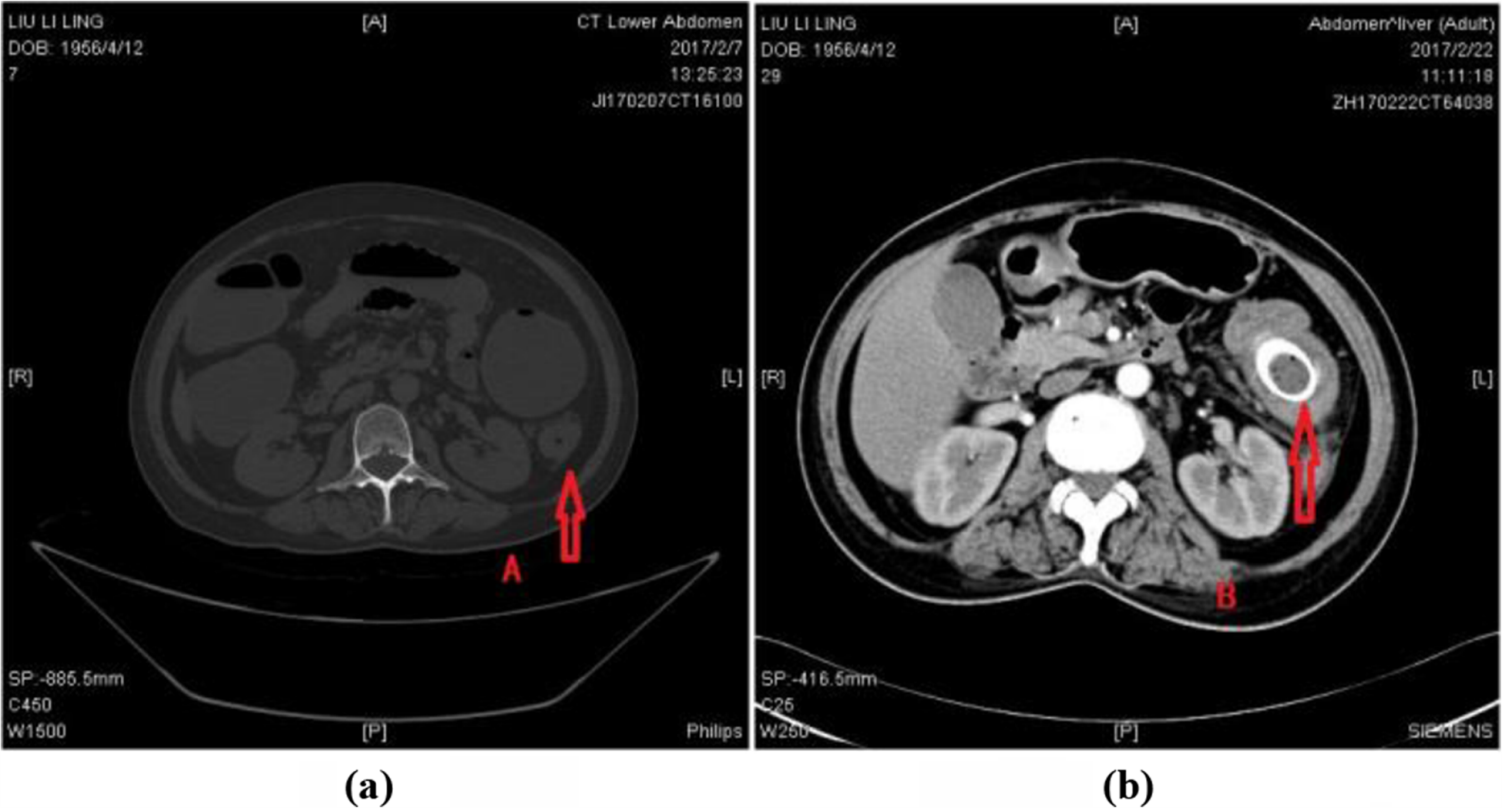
Axial computed tomography images demonstrating an obstructing carcinoma with gross proximal colonic dilatation (a). After successful decompression by stenting (b)
